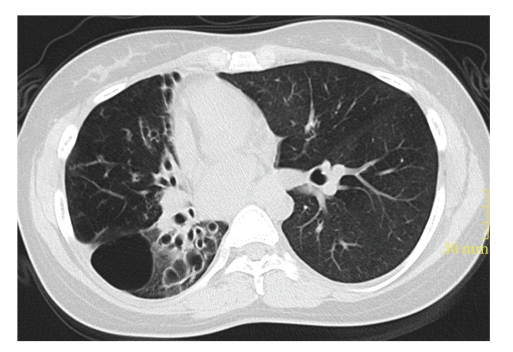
Preoperative chest CT scan revealing right accentuated severe bronchiectasis accompanied by pulmonary emphysema.
